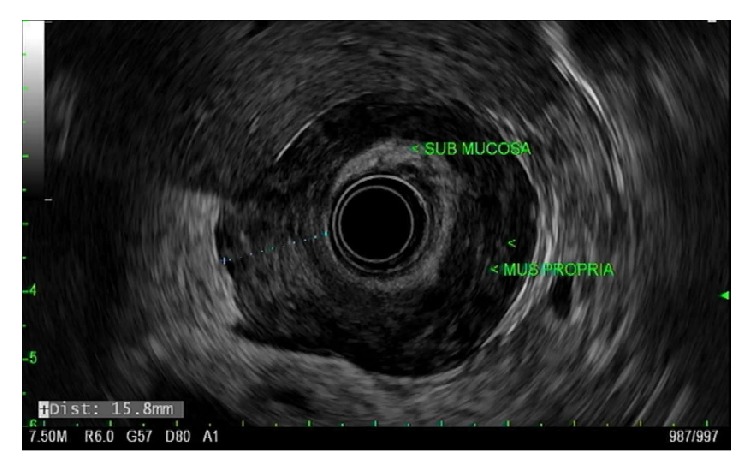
Thickened gastric muscularis propria layer on endoscopic ultrasound.
